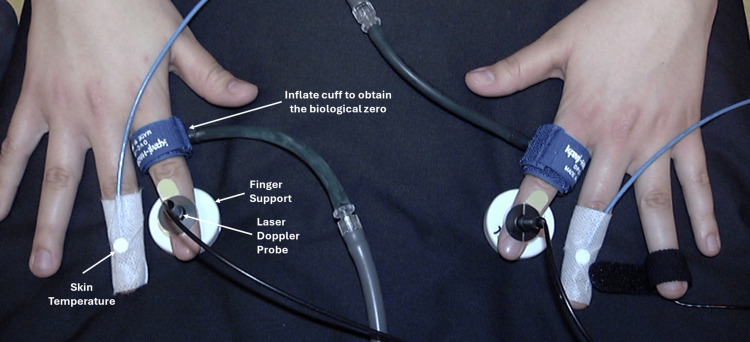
Setup for bilateral finger dorsum laser Doppler-determined IGVR The target fingers are supported for comfort on a surface that could be heated, if desired. Basal skin temperature may be recorded with a suitable thermistor on an adjacent finger, as shown. The cuff at the base of the fingers is inflated at the end of the experiment to record the LDF BZ. The BZ is the value of the laser Doppler output when blood flow is forced to zero by the cuff inflation. BZ, biological zero; IGVR, inspiratory gasp vascular reflex; LDF, laser Doppler flowmetry This previously unpublished figure is provided courtesy of Harvey N. Mayrovitz.
